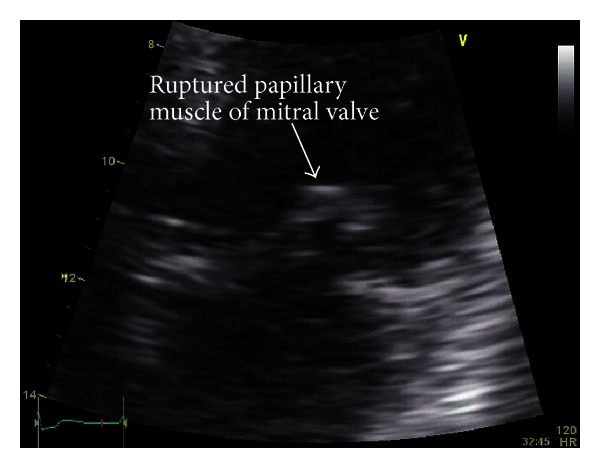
Cardiac echography, apical four chambers view. Echodense prolapsing 10 mm mass attached to the anterior mitral valve leaflet leading to severe mitral regurgitation.
